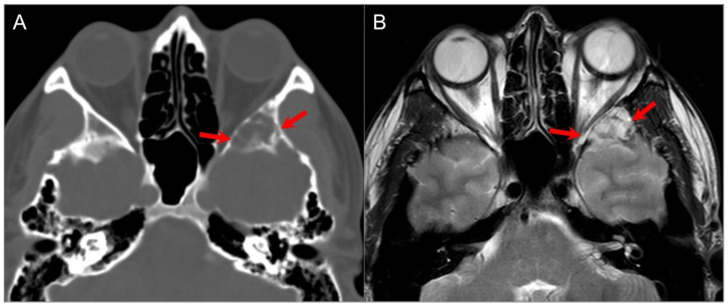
Sphenoid wing cephalocele. (A) Axial CT image reveals a lobulated, well-defined lucent lesion in the left greater sphenoid wing with bony scalloping and thin internal septations (red arrows). (B) T2W axial MR image shows CSF isointense signal within the lesion with linear hypointense septations.
